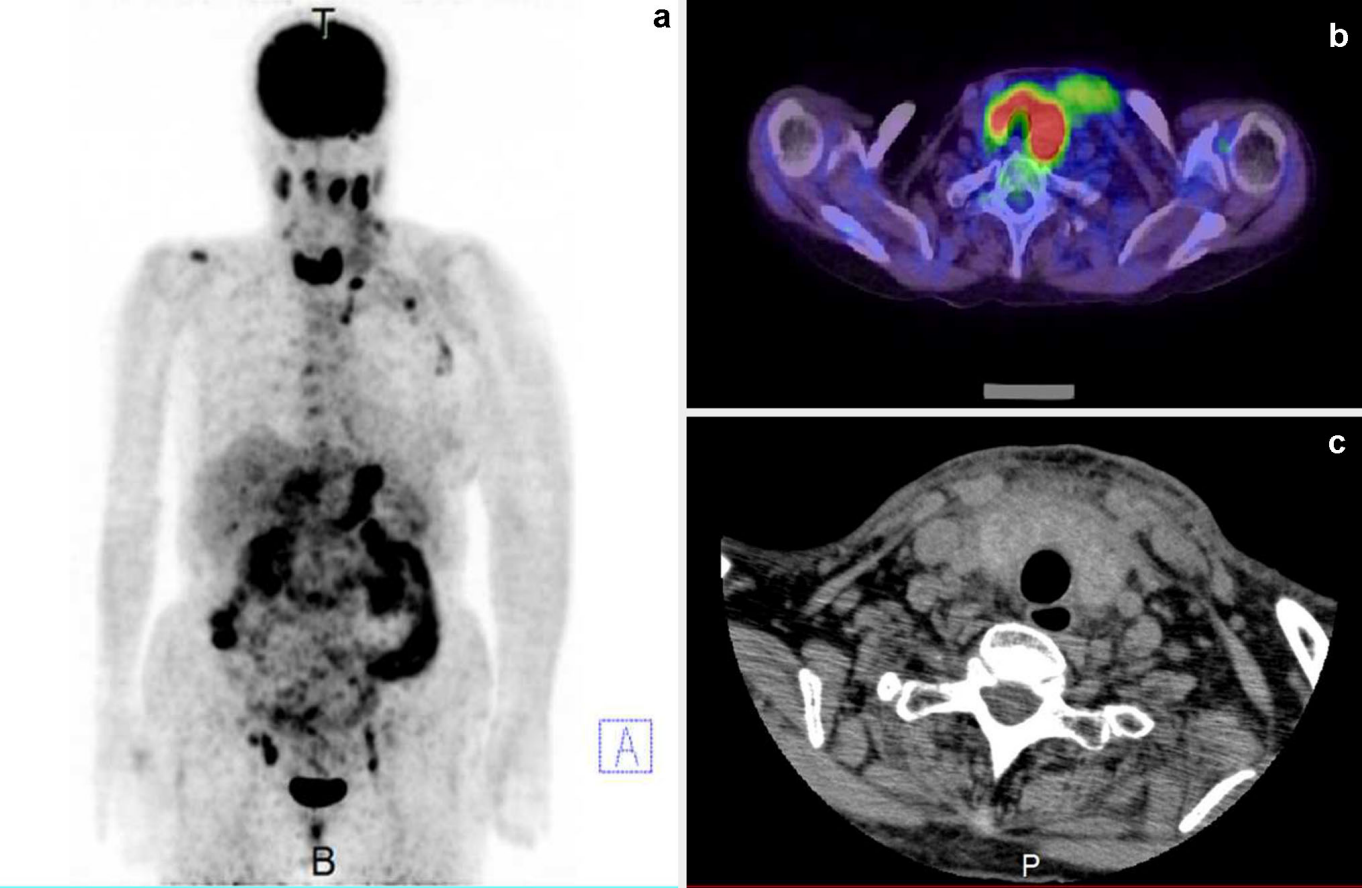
a Coronal maximum intensity projection (MIP) of 18F-FDG PET imaging before admission. Accumulation was found in the stomach, in the right hepatic lobe, in the extensive lymph node metastases, and in the whole thyroid gland. b Transverse section of the thyroid on 18F-FDG PET/CT imaging before admission. Diffuse uptake in bilateral thyroid lobes was observed. c Transverse section of the thyroid on CT imaging after admission. The thyroid gland was diffusely swollen. Its size enlarged and its CT value decreased after hospitalization. In addition, the adipose tissue concentration in the surrounding area increased
